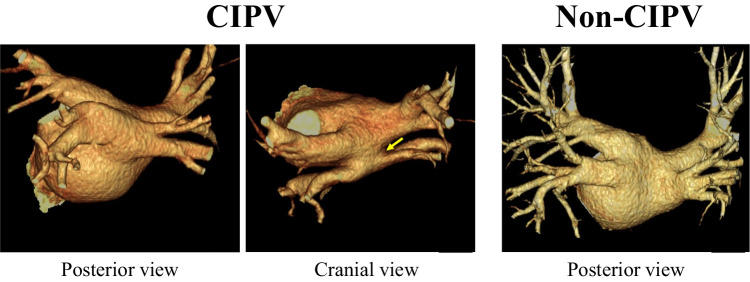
3-dimensional computed tomographic images of the left atrium (LA) of confluent inferior pulmonary veins (CIPV) and non-CIPV cases. In CIPV cases, the left and right inferior pulmonary veins had continuity behind the LA posterior wall with a common trunk (yellow arrow) connecting to the LA
